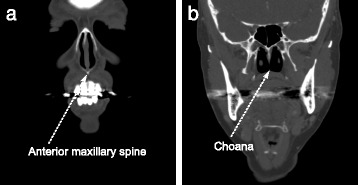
Bone landmarks in CT scans. a Maxillary spine landmark b Choana landmark
